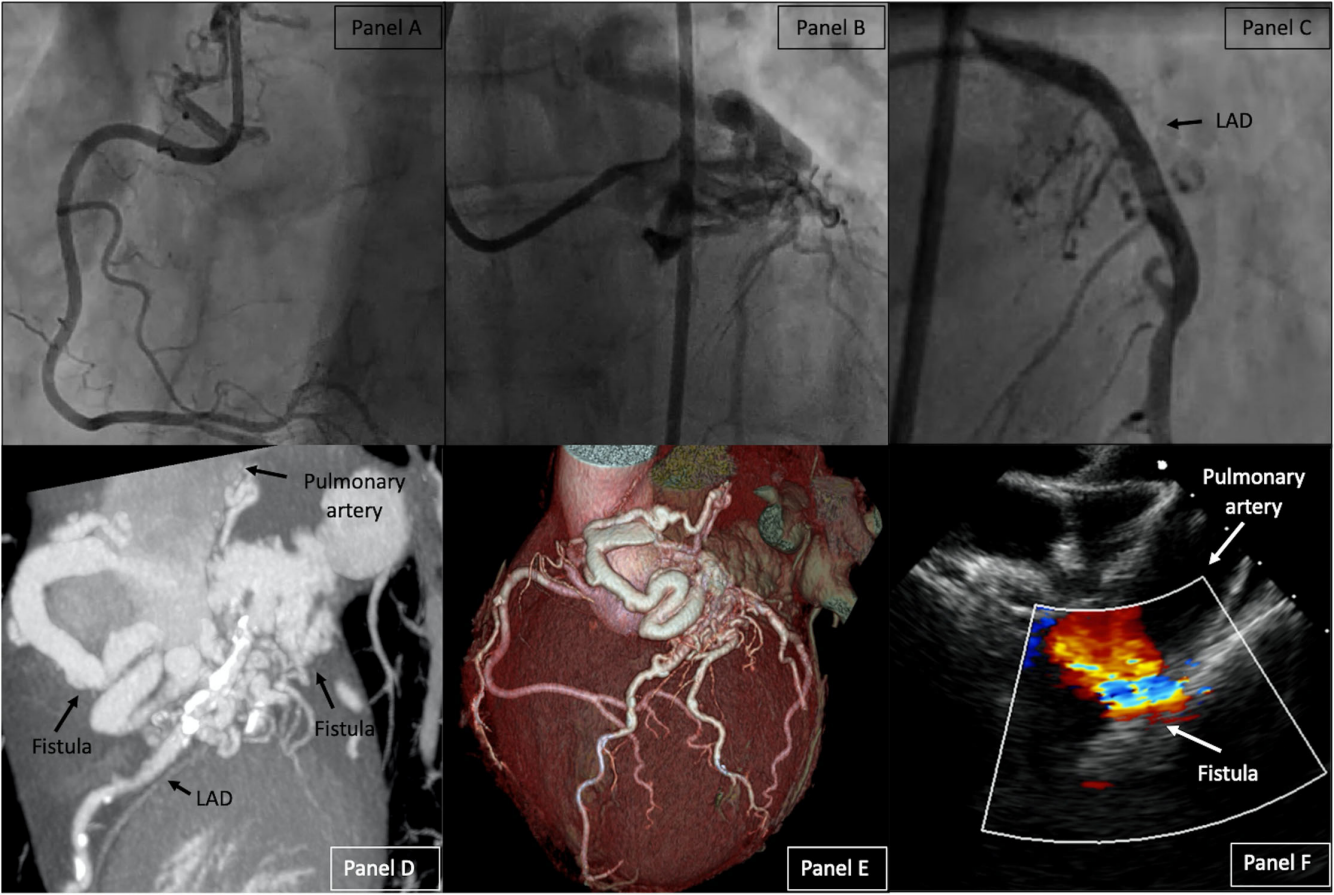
Right Coronary Artery (RCA) with the right part of the fistula (A), Left Coronary Artery (LCA) with the left part of the fistula (B), Selective LAD injection (C). Baseline CCT with 2D Maximum intensity projection of the fistula (D) and 3D volume rendering of the heart (E). TOE before fistula closure (F). LAD, left anterior descending artery; CCT, *cardiac CT*; TOE, *transoesophageal echocardiogram*.
